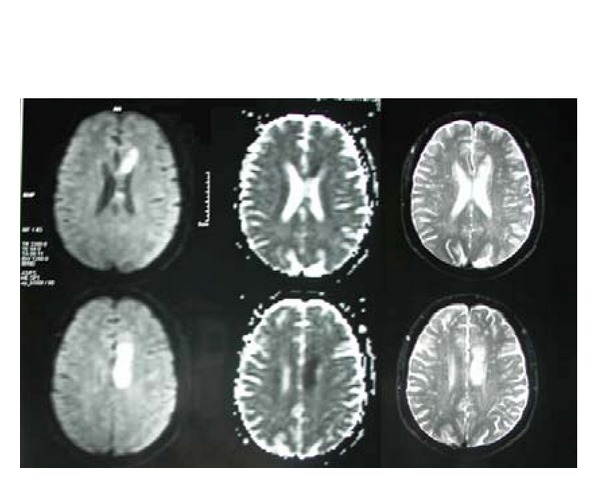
The first MRI shows an infarct in left centrum semiovale near the left lateral ventricle with mismatch in DWI and ADC map imaging and also multiple lacunar infarcts.
